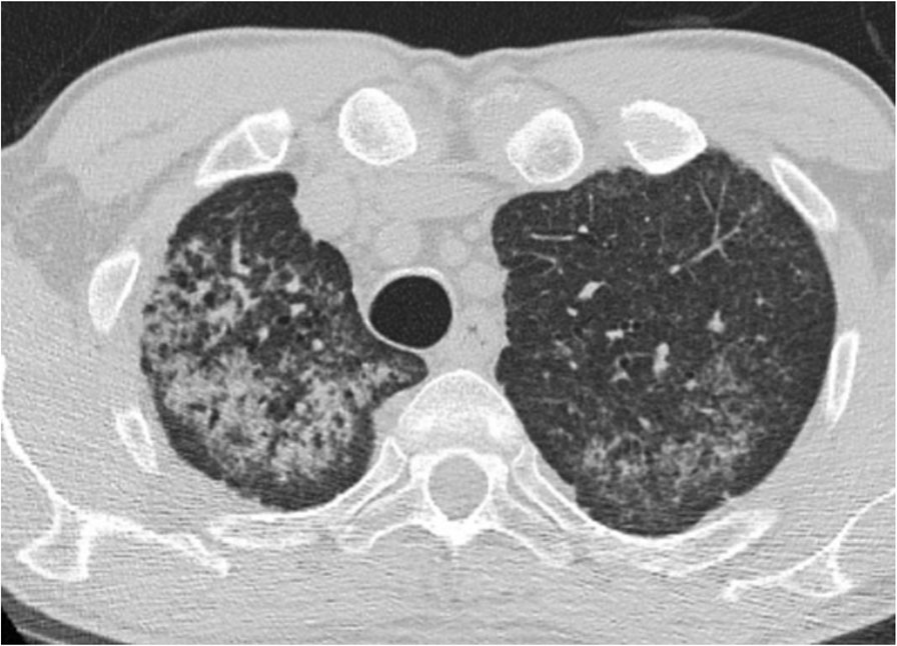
High resolution CT scan showing extensive peribronchiolar consolidations, almost exclusively involving the upper zones of the lungs (mainly the right upper lobe). The pleura and subpleural parenchyma is almost always spared
